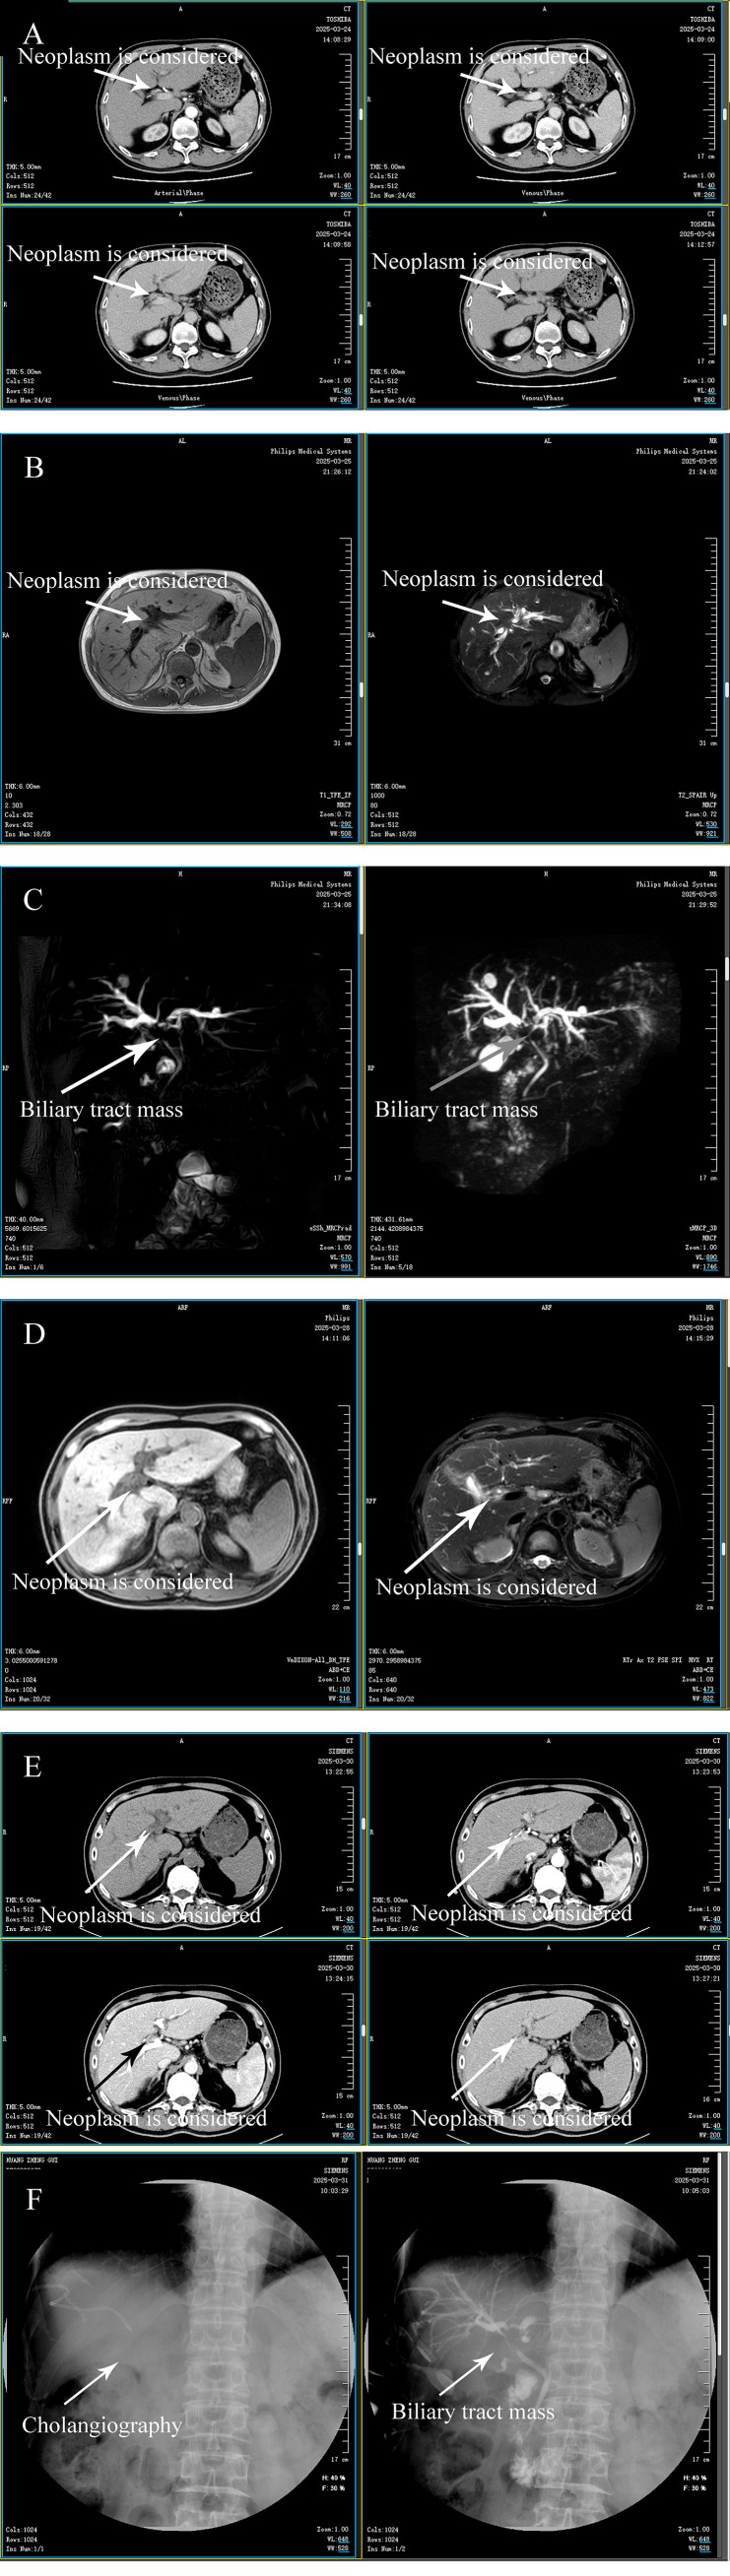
Imaging findings of IGG4-related cholangitis. (A, B) Plain and enhanced CT examinations of the liver, gallbladder, spleen, and pancreas, with neoplasms indicated. (C, D) MRCP (magnetic resonance cholangiopancreatography) of the pancreaticobiliary system, showing a biliary tract mass. (E, F) Plain and enhanced MR examinations of the liver, with neoplasms indicated.
